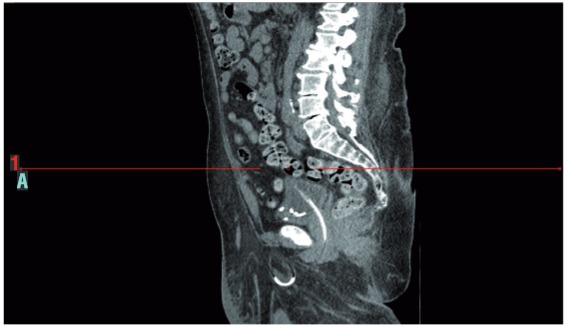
In this sagittal section of the CT-KUB, the left ureter, as outlined by the stent, can be seen passing through the neck of the hernia sac.
